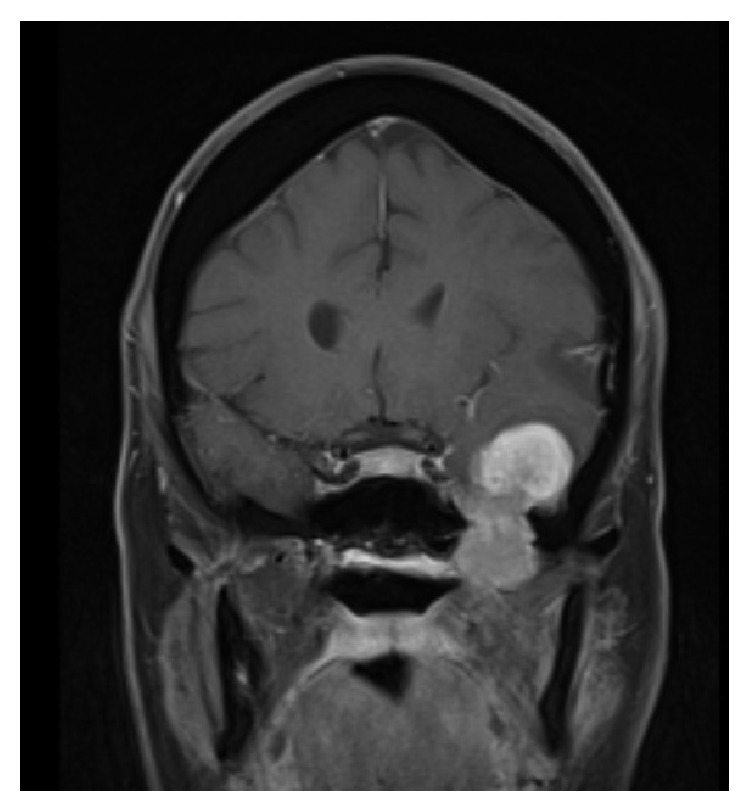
Coronal T1-WI MRI demonstrating middle cranial fossa tumor extending to the infratemporal region.
